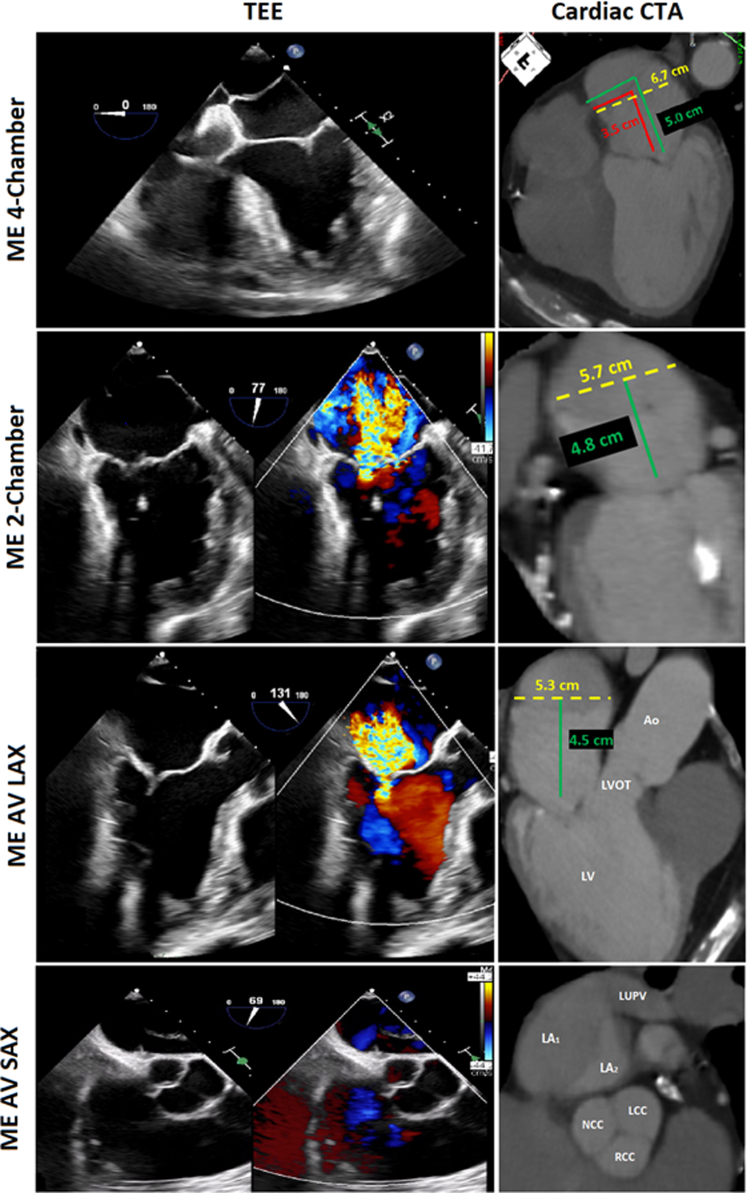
Preprocedural Cardiac CT with Coregistered TEE Images Various configurations of the left atrial membrane with coregistered TEE measurements revealing a small window for posterior puncture placed above the membrane. The membrane attaches anteriorly on the interatrial septum, connecting the left upper pulmonary vein to the anterior region of the fossa ovalis. Yellow dotted lines delineate LA width. Red line delineates the membrane height from the mitral valve. Green delineates the planned site of puncture of the interatrial septum. AV = aortic valve; CT = computed tomography; LA = left atria; LAX = long-axis; LCC = left coronary cusp; LV = left ventricle; LVOT = left ventricular outflow tract; LUPV = left upper pulmonary vein; ME = mid-esophageal; NCC = noncoronary cusp; RCC = right coronary cusp; RV = right ventricle; SAX = short-axis; TEE = transesophageal echocardiography.
